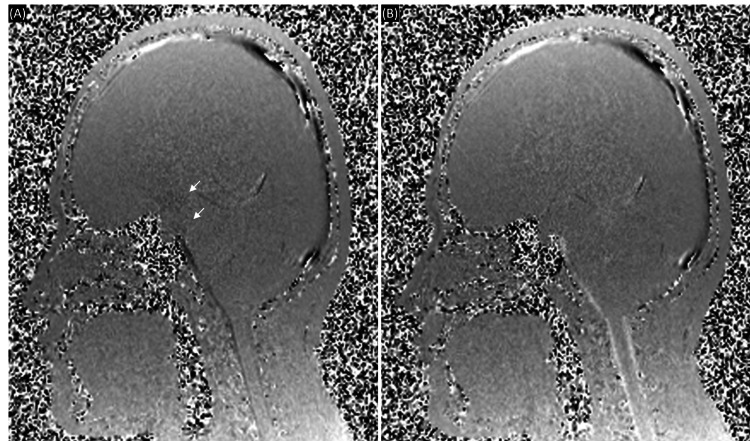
Cine phase-contrast MRI images of the patient's head in sagittal view. The pulsatile flow of cerebrospinal fluid (CSF) is shown in the images. (A) The cranial flow of CSF during diastole is demonstrated in black. (B) The caudal flow of CSF during systole is demonstrated in white. White arrows indicate the flow of CSF through spontaneous third ventriculostomy.
